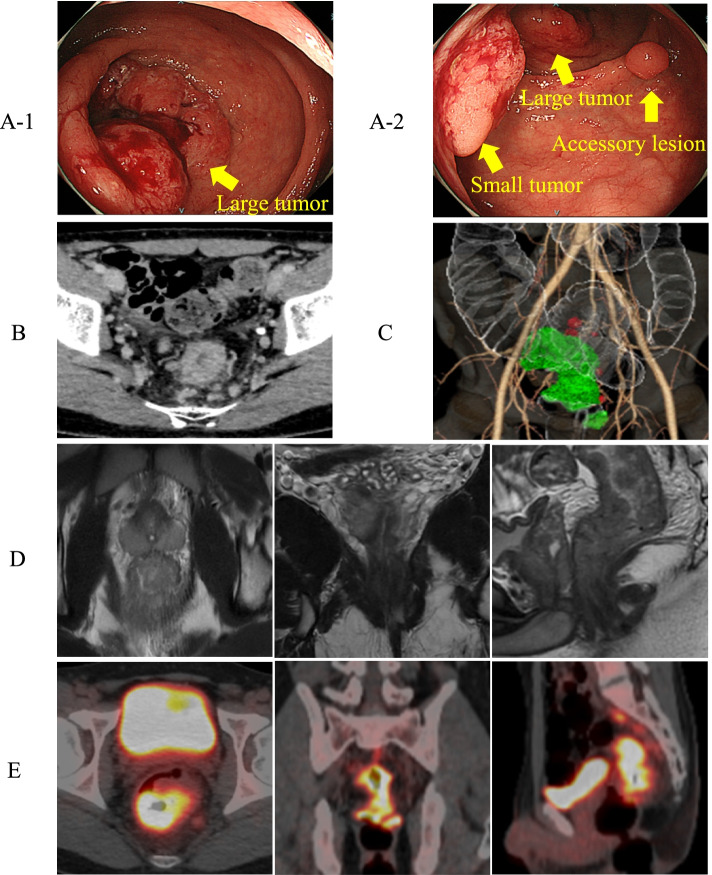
Pretreatment imaging findings. A CS images. Two tumors and an accessory lesion between them were identified in the rectum. The large main tumor was a circumferential advanced cancer and located on the second Houston valve (5 cm from anal verge), causing severe stenosis (A1). The small tumor was an early cancer 2 cm in diameter and it was about 0.5 cm from the dentate line (2.5 cm from the anal verge ). The accessory lesion was an adenoma 0.3 cm in diameter (A2). B Axial CT image. There was misty mesentery surrounding the large tumor and many enlarged pararectal lymph nodes. C CT colonography with three-dimensional reconstruction. D MRI images. The large tumor was located at the peritoneal reflection, with irregularity of the serous surface. The anterior border of the tumor was close to the prostate. E PET-CT images. ^18^F-FDG uptake was detected in tumors and lymph nodes. SUVmax: large tumor, 16.07; small tumor, 13.41
